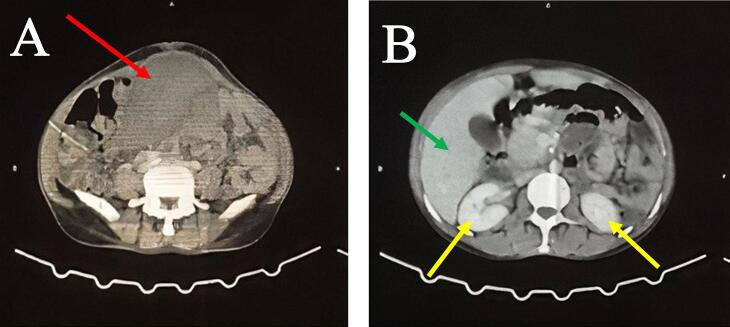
The axial CT image from (A) and (B) reveals that the spleen (red arrow) is not located in its usual position. It is situated at a lower level of the kidneys (yellow arrow) and in the pelvic cavity. Liver in (green arrow). (For interpretation of the references to colour in this figure legend, the reader is referred to the web version of this article.)
